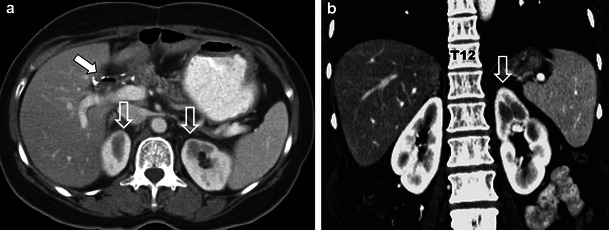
A 49-year-old woman who underwent pylorus sparing Whipple’s procedure and RT (5,940 cGy) for ampullary cholangiocarcinoma demonstrates typical findings of chronic radiation nephropathy on 3-year follow-up imaging. Axial (a) and coronal (b) contrast-enhanced CT (CECT) images demonstrate focal atrophy of the medial upper poles of both kidneys with a smooth contour (*open white arrows*). Note regional osteopenia in L1-L3 in (b) and post-operative pneumobilia in the bile duct (*solid white arrow* in a) indicating patent choledochojejunostomy (not shown)
